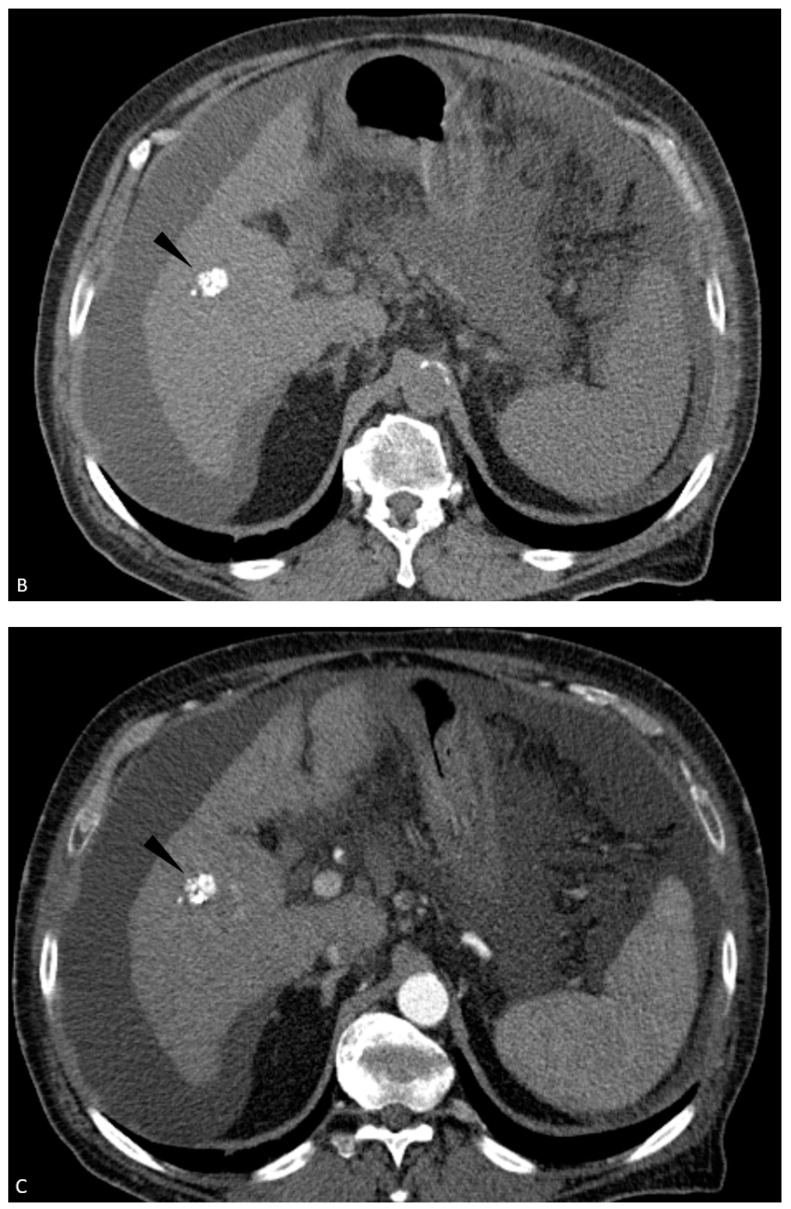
A 63-year-old male with cirrhosis due to MASH. (A) CEUS and accompanying grayscale image at 14 s following administration of Lumason^®^. Black arrow corresponds to internal enhancement thought to represent residual disease. Enhancing area demonstrated washout on delayed images. (B,C) Corresponding same-day CT showing no viable, enhancing lesion (black arrowheads), (B) non-contrast CT, (C) late arterial-phase CT.
